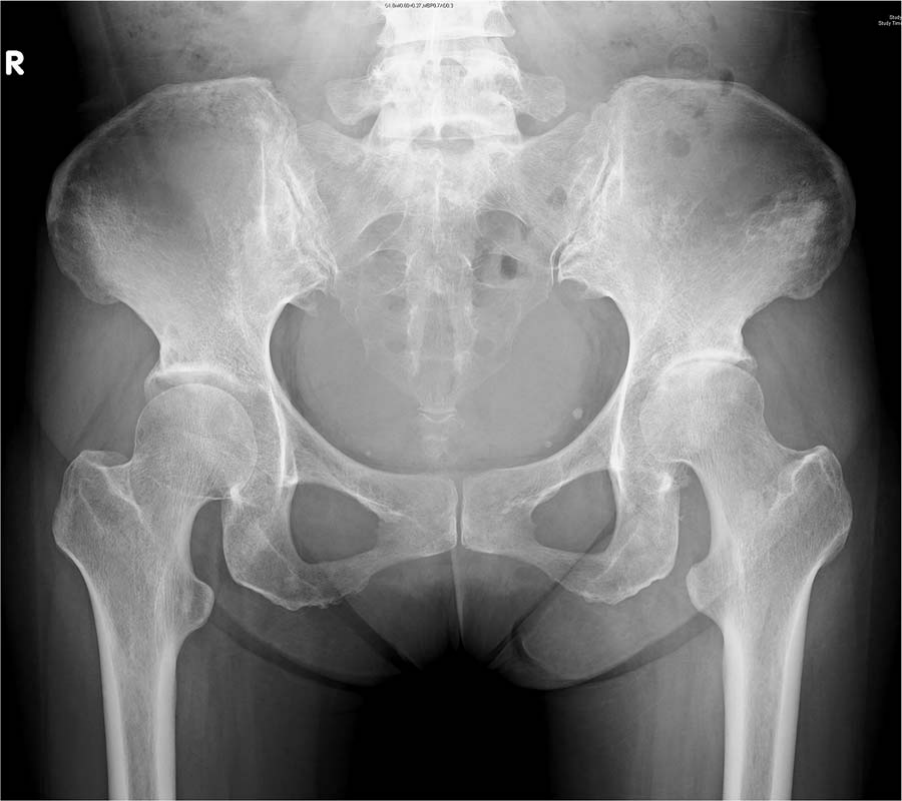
Pelvic radiograph of a 44-year-old female showing symmetric left hip joint space narrowing, indicative of hip septic arthritis.
